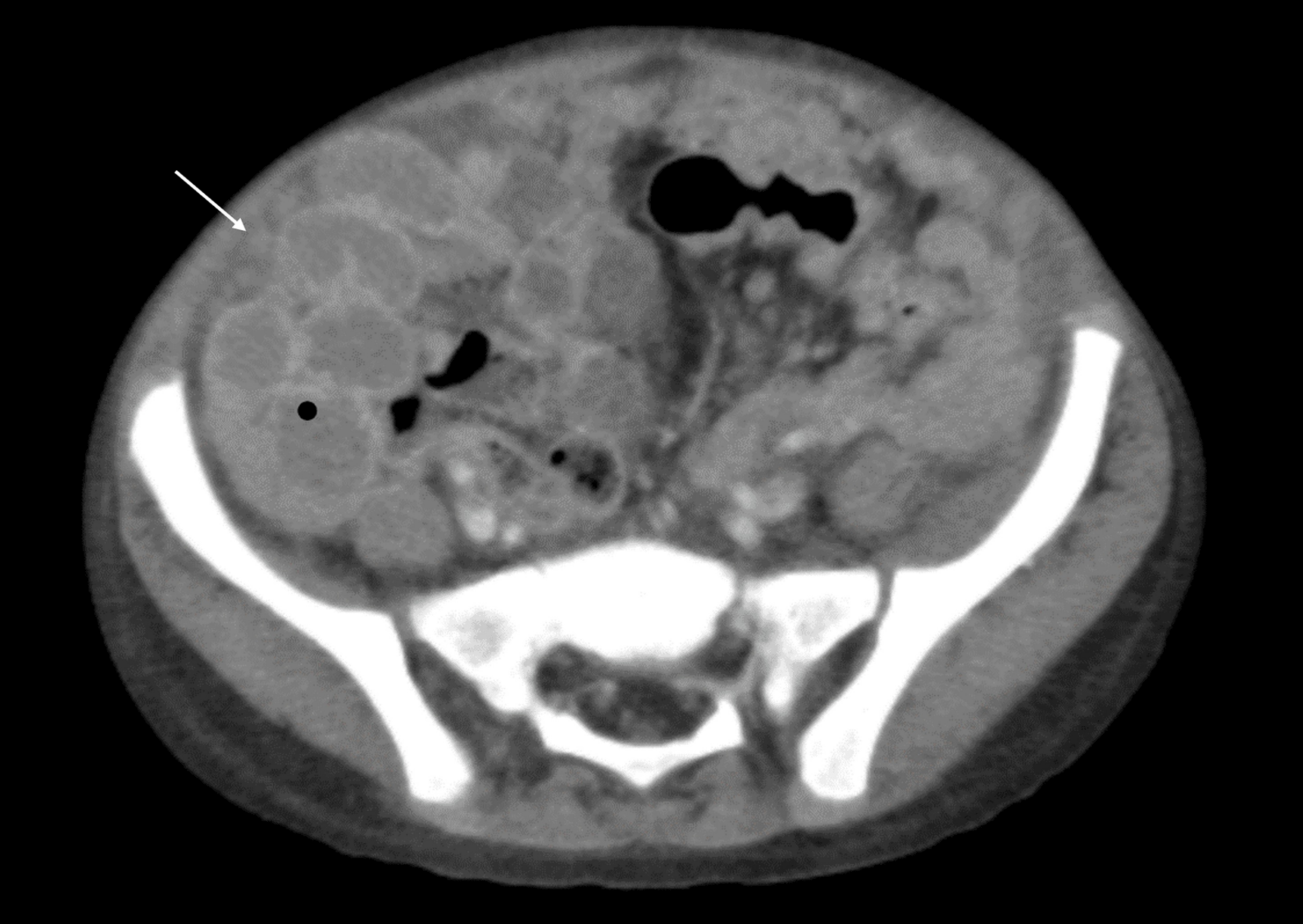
CT image (19-02-2024) showing peritoneal nodules. CT, computed tomography.
